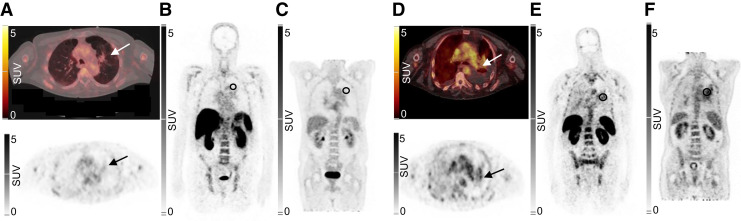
Uptake of [^68^Ga]Ga-NOTA-anti-CD206-sdAb in patient 1 (A–C) and patient 2 (D–F), both with non–small cell lung carcinoma and residual lesion in lung region during treatment. (A and D) Uptake in lung lesion (arrow) on PET/CT (top) and PET (bottom) images 90 min after injection. Coronal PET images show lesional uptake, indicated by ellipsoid in left lung, for [^68^Ga]Ga-NOTA-anti-CD206-sdAb (B) and [^18^F]FDG performed 15 wk after study participation (C) of patient 1, who showed long-term disease remission. Coronal PET images show lesional uptake, indicated by ellipsoid in left lung of patient 2, for [^68^Ga]Ga-NOTA-anti-CD206-sdAb (E) and [^18^F]FDG performed 11 wk later (F). This patient showed disease progression on these [^18^F]FDG PET/CT images.
